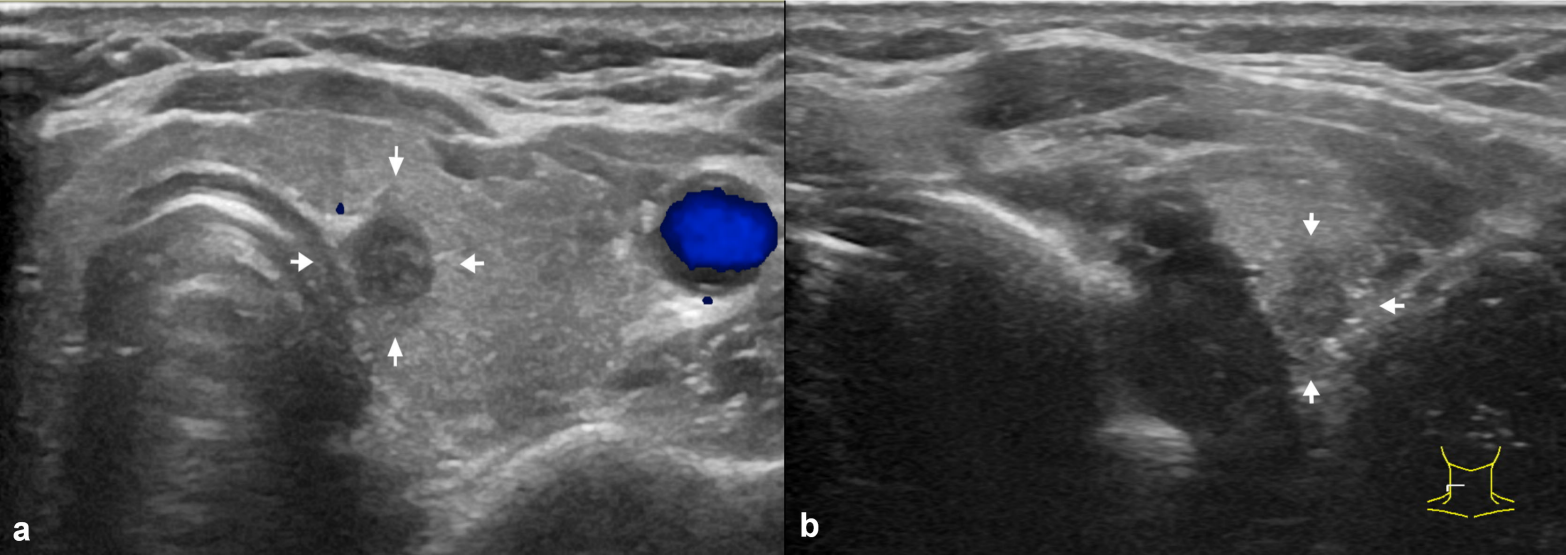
Effective isolation of ultrasound extrathyroidal extension (uETE) nodules. State of the sample before (a) and after (b) hydrodissection.
